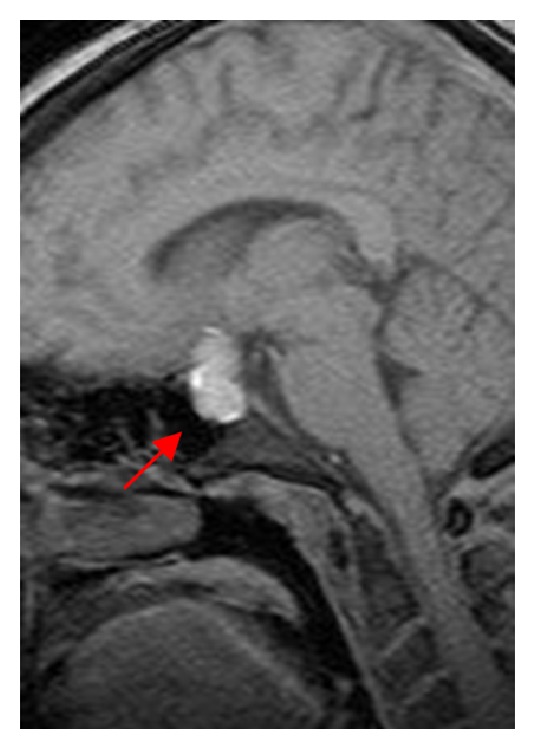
Sagittal T1 MRI of brain without contrast shows area of hyperintensity within the sella and suprasellar regions (red arrow). This likely represents pituitary apoplexy within the known macroadenoma.
